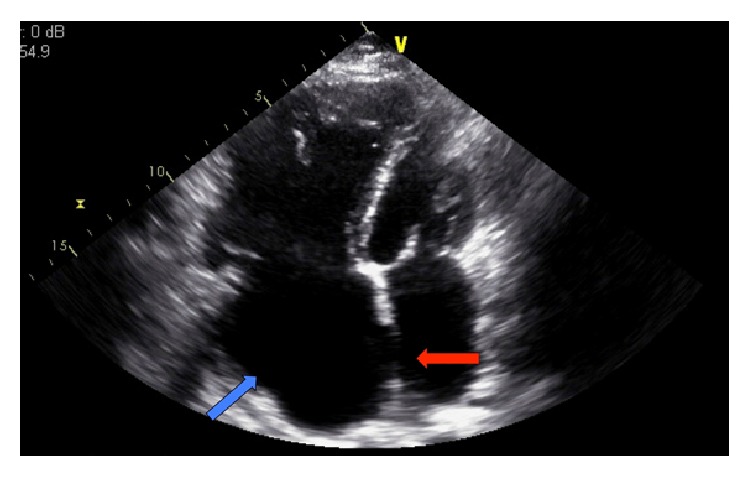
Echocardiography showing enlarged right ventricle and right atrium (blue arrow) with sinus venosus defect (red arrow).
